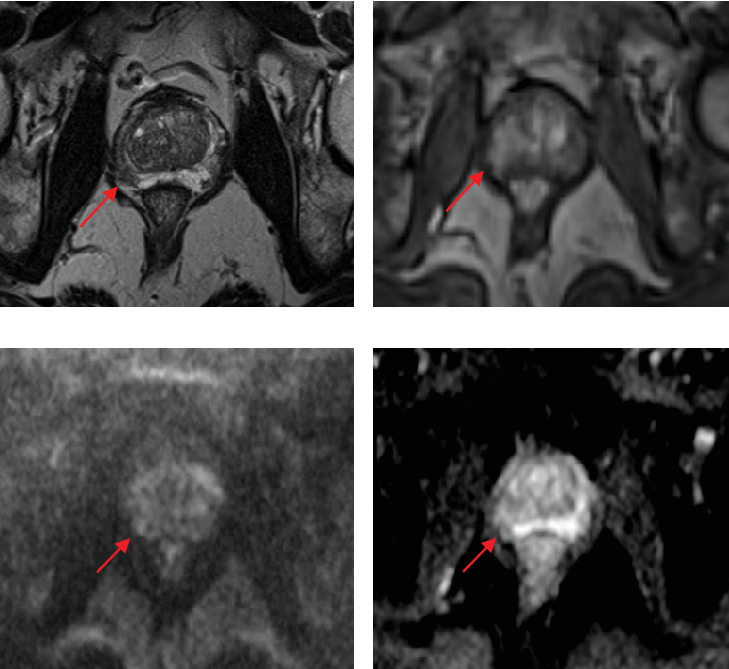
Representative MRI of the right base posterior peripheral zone lesion measuring 0.6 × 0.4 cm (red arrows). This lesion was assigned a PI-RADS 3 score. (a) T2-weighted, (b) postcontrast T1-weighted, (c) diffusion-weighted imaging (DWI), and (d) apparent diffusion coefficient (ADC).
